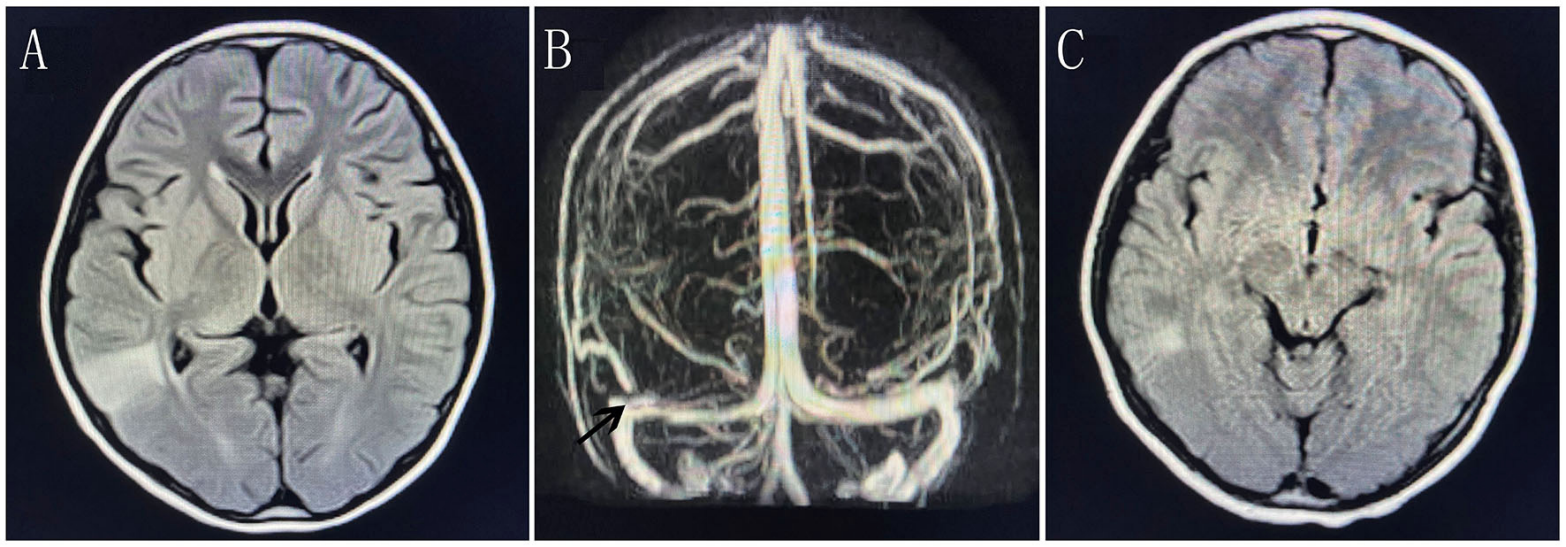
Brain magnetic resonance imaging at follow-up. (A) Flair sequence before discharge. (B) MRV scanning at four-month follow-up. The black arrow indicates the interruption of blood flow at the junction of the right Labbe vein and right transverse sinus. (C) Flair sequence at four-month follow-up.
